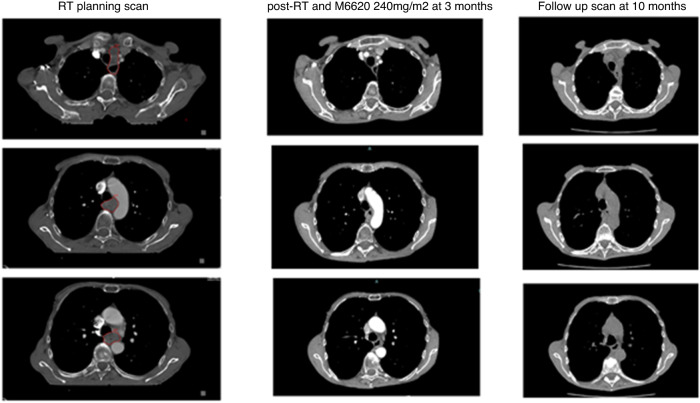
Patient case: 3 axial CT slices at radiotherapy planning, 3 months of treatment w/ berzosertib, 10-month follow-up.
